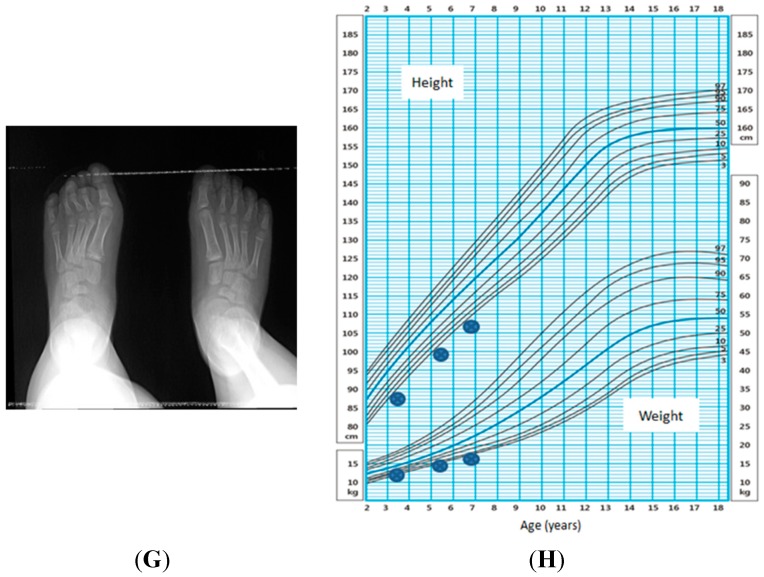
Physical characteristics of the proband. Patient’s eyes are covered for reasons of confidentiality. Typical facial dysmorphic features can be observed in (A,B), including highly arched eyebrows, a broad nasal bridge, beaked nose with the nasal septum extending well below the alae, a pouting lower lip, and mild micrognathia. Dental crowding is shown in (C); The hand and feet abnormalities are shown in (D,E), showing short and broad big toes and thumbs, as well as mild clinodactyly on the 5th digit of both hands. The 2nd, 3rd, 4th digits of the right hand had short distal phalanges and the 3rd, 4th digits of the left hand had broad distal phalanges. The X-ray of the left hand shows delayed carpal bone ossification and prominent thickening of the soft tissue of all fingers (F); The X-ray of the feet shows bilateral pes varus deformities and abnormalities in the physes of big toe metatarsal bones (G); Growth curve (H) shows marked growth retardation in the proband (blue dot) superimposed on the normal growth curve of Korean girls of age 2 to 18 (black line). The growth curve shows the height and weight changes of the patient over time. These values never exceeded the third percentile since birth. Data for normal growth curve came from the Korea Center for Disease Control and Prevention.
